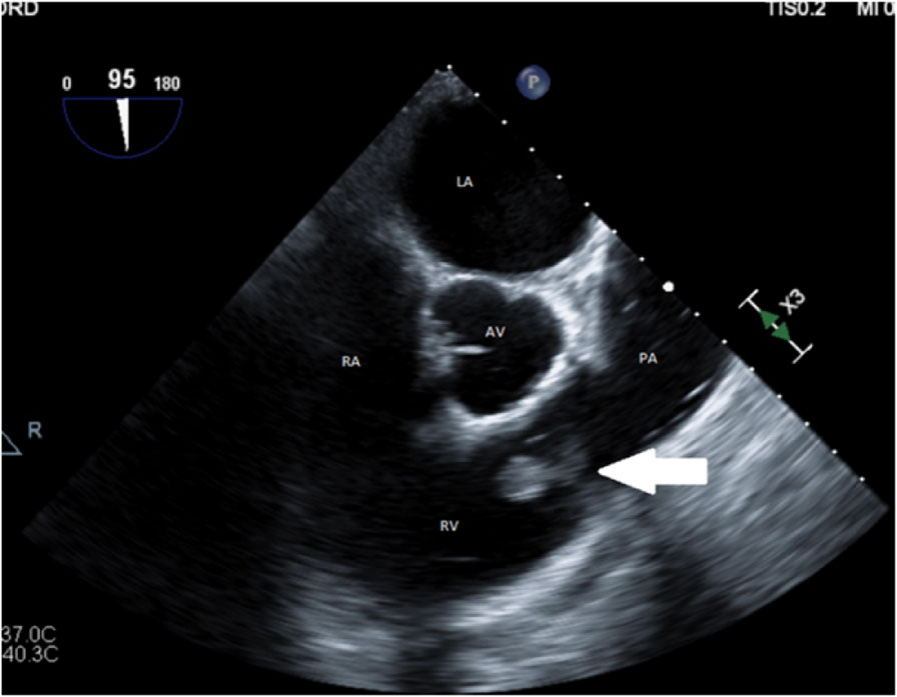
Transesophageal echocardiography showing vegetation (*arrow*) between right ventricle (RV) and pulmonary artery (PA). *AV* Aortic valve LA Left atrium, RA Right atrium
